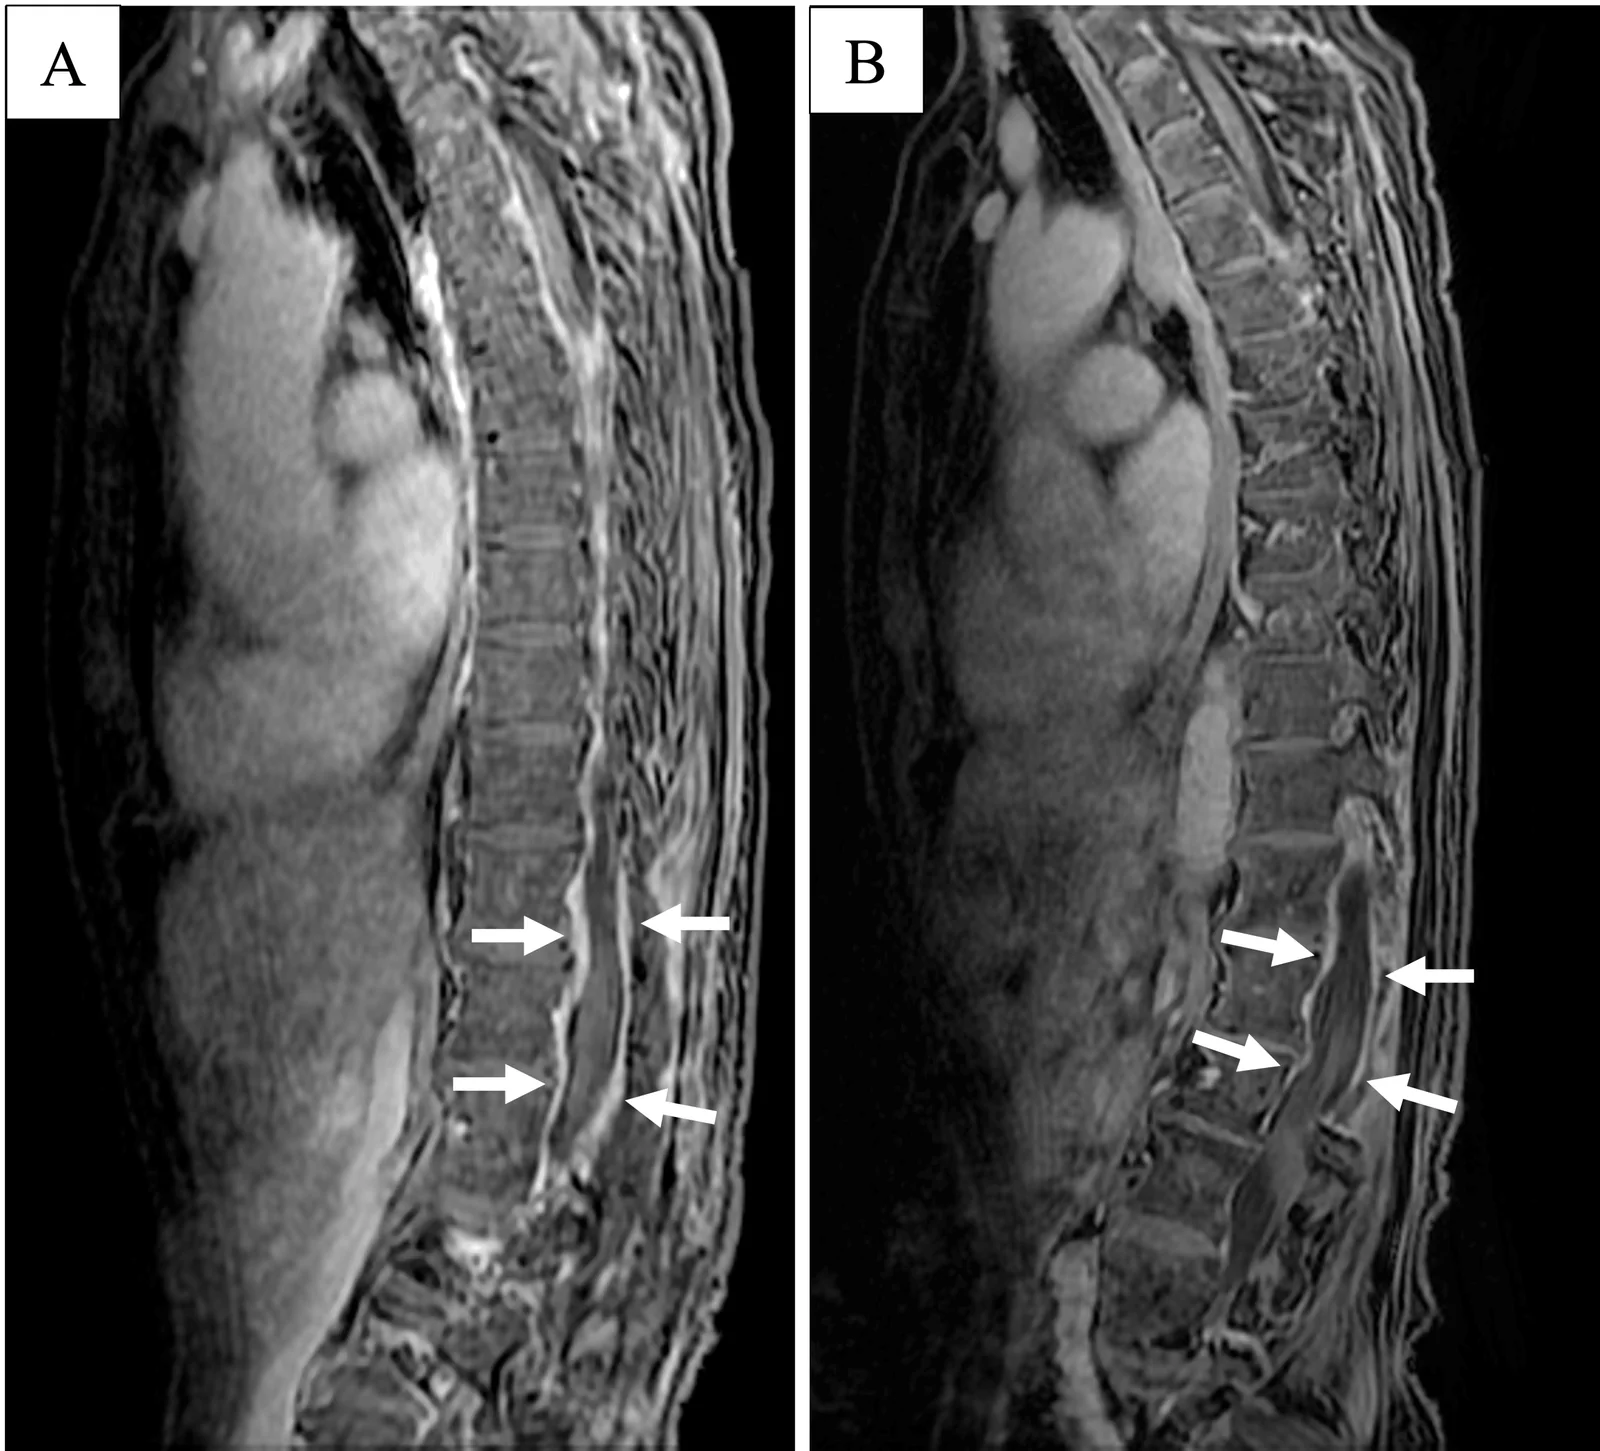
Follow-up gadolinium-enhanced MRI. (A) On day 2 after the initiation of intravenous hydrocortisone, a contrast-enhanced sagittal T1-weighted image shows a reduction in dural thickening (white arrow). (B) On day 34 after initiating treatment, contrast-enhanced MRI shows almost complete disappearance of the dural thickening (white arrow).
